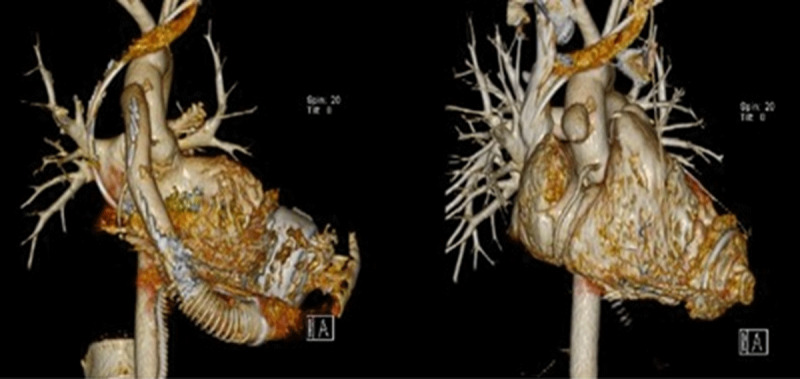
Pre- and postoperative computed tomography scans. Residual left ventricular assist device fixation ring present with hemostatic synthetic plug and notable absence of left ventricular thrombus.
